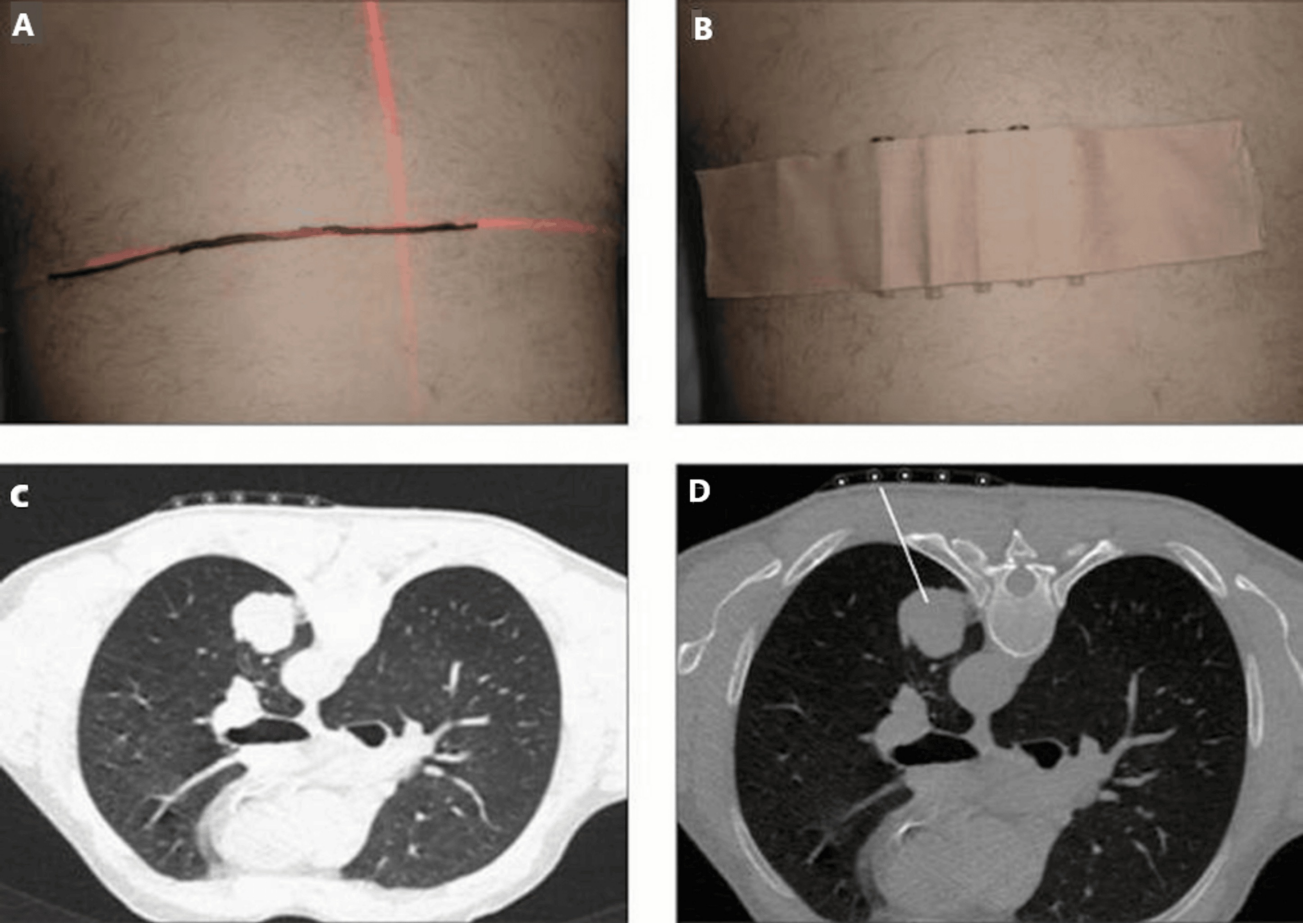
CT-guided lung biopsy process. The lung lesion is identified and marked on the patient's chest, as shown in (A). Radiopaque markers can be applied to increase the accuracy of biopsy, as seen in (B), although not always necessary. A scan is taken again following the application of the radiopaque marker (C), and the shortest distance path determined is determined. The needle is then inserted along that path to biopsy the target lesion (D).
